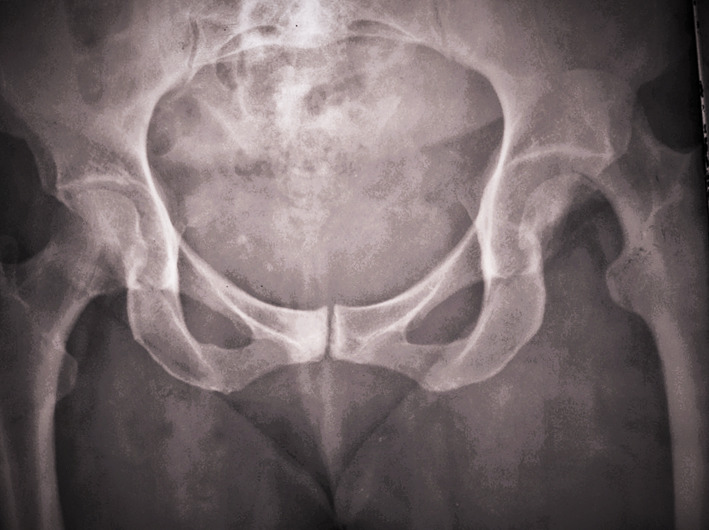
Preoperative radiograph of a female 35-year-old patient with left hip dislocation and fracture of the femoral head (Pipkin type 1 fracture)
